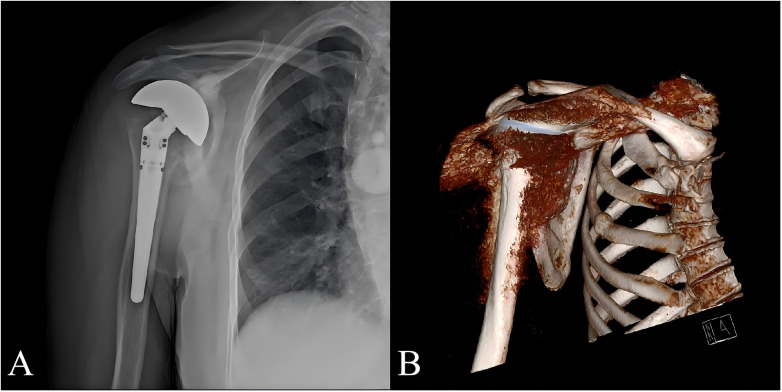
One-year postoperative anteroposterior shoulder X-ray (A) and 3D reconstruction (B) showing the prosthesis securely in place.
